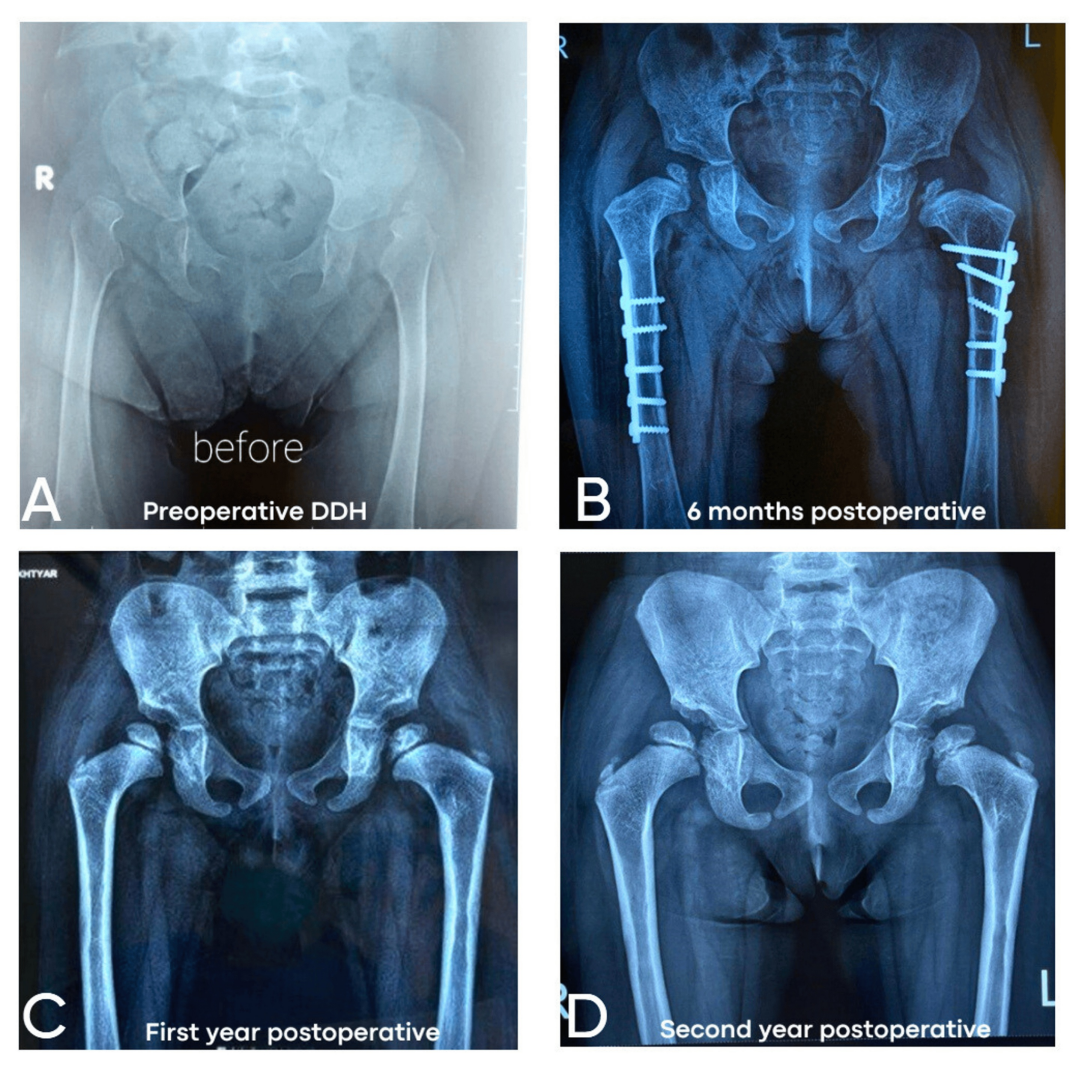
Sequential radiographic assessment of a patient with developmental dysplasia of the hip undergoing arthroscopic-assisted reduction. (A) Preoperative radiograph. (B) Six-month postoperative radiograph: the fixation hardware is visible, indicating derotational femoral osteotomy to improve excessive femoral anteversion. Early signs of remodeling of the acetabular roof and reduction in the acetabular index are evident. (C) One-year postoperative radiograph: the acetabular roof shows further remodeling with improved femoral head coverage. The acetabular index has decreased, suggesting progressive correction of dysplasia. (D) Two-year postoperative radiograph: bone healing around the osteotomy site is complete, and the absence of avascular necrosis is evident, indicating a favorable outcome.
